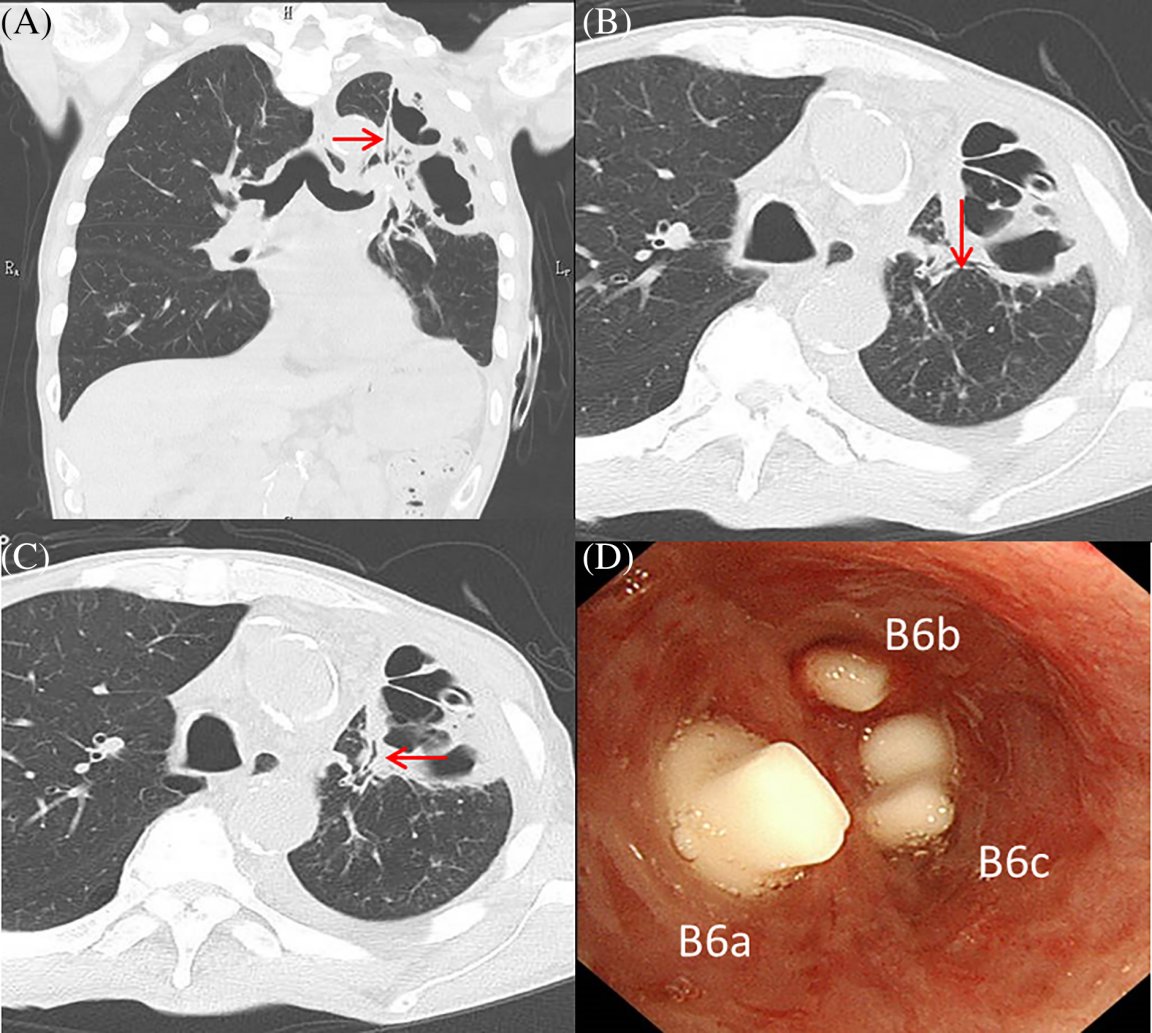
Chest computed tomography imaging shows the target bronchi for bronchopleural fistulas. (A) B6a; (B) B6b (C) B6c; (D) bronchoscopic findings after bronchial occlusion with endobronchial Watanabe spigots.
